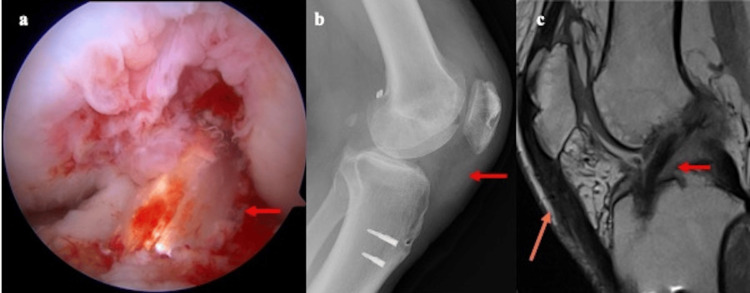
Intraoperative finding and postoperative images (a) Single bundle ACL reconstruction using the ST. (b) Postoperative radiograph. Insall-Salvatti ratio: 1.1. (c) Postoperative MRI. Reconstructed PT (indicated by the red arrow) and ACL (indicated by the orange arrow) had good continuity and density. ACL: anterior cruciate ligament, MRI: magnetic resonance imaging, PT: patellar tendon, ST: semitendinosus tendon
